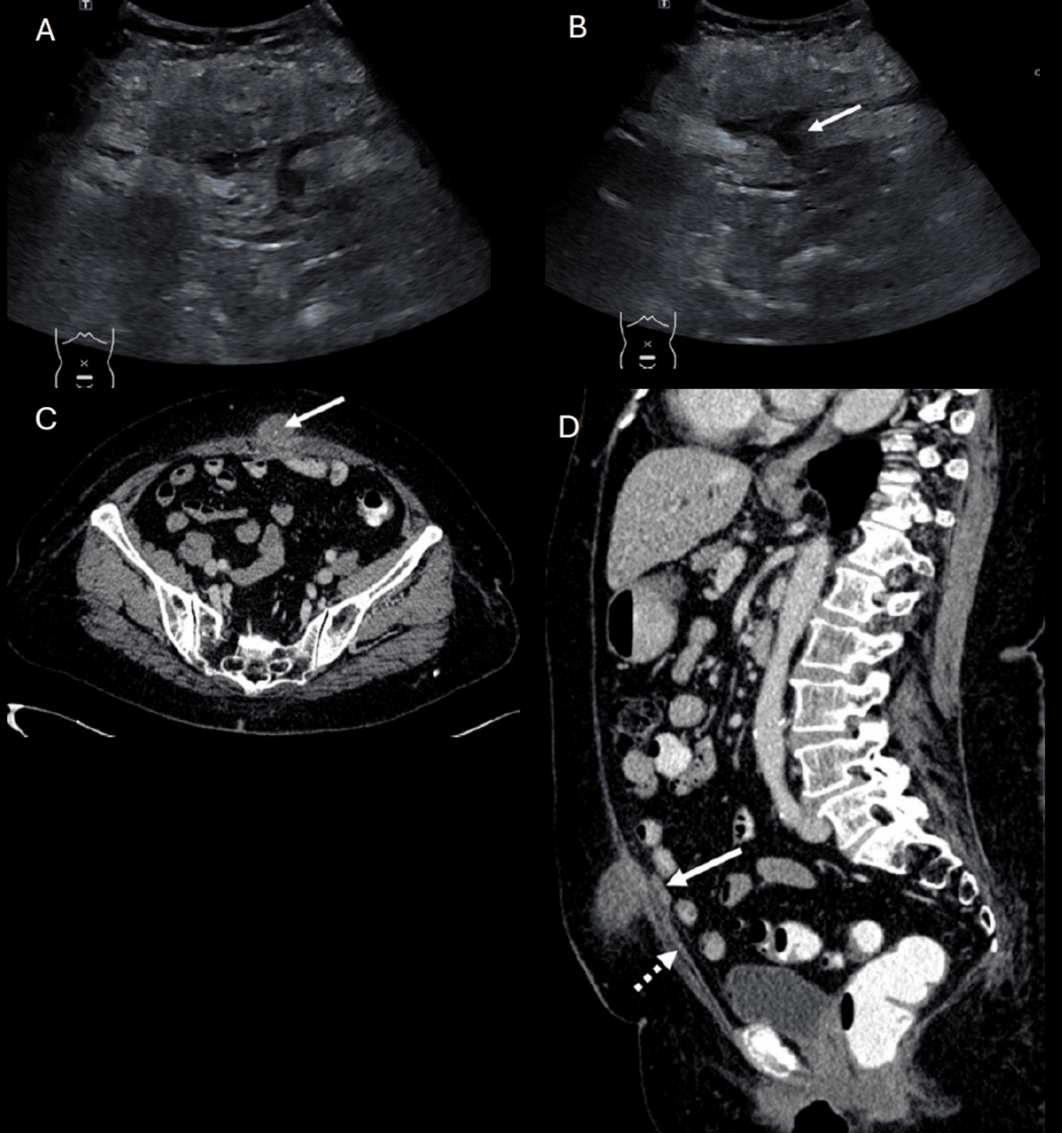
Ultrasound of the anterior abdominal wall (A and B) reveals an avascular, poorly defined, and heterogeneous echogenic subcutaneous collection in the lower anterior abdomen, measuring 2.6 x 5.9 x 4.6 cm (AP x W x CC), with trace amounts of liquefied components (arrow, B). The overlying subcutaneous tissue is notably thickened and oedematous. Subsequent axial contrast-enhanced CT of the abdomen (C) corroborates the ultrasonographic findings, further elucidating the heterogeneous subcutaneous lesion and identifying a small calcification focus within it (arrow, C), along with subcutaneous fat stranding. Additionally, the sagittal contrast-enhanced CT view (D) depicts the thickened proximal attachment of the medial umbilical ligament (dash arrow, D) and urachal remnant (arrow, D), providing further characterization of the abnormality. AP = anteroposterior; W = width; CC = craniocaudal.
